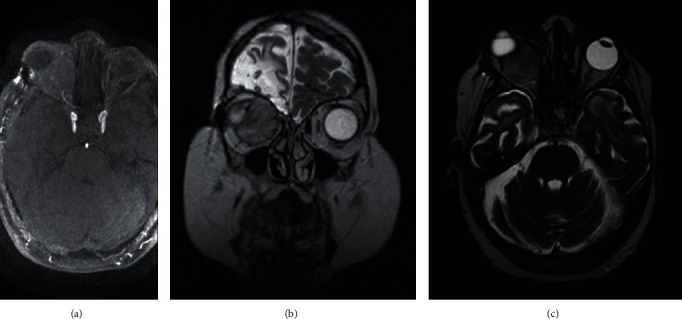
Case 4. (a) CT imaging with a great lesion, hyperdense morphology appears in the right intraconal region. The lesion imprints on the back of the globe, as well as the optic nerve and internal rectus muscle, with close contact with the external rectus muscle. (b, c) MR imaging, coronal view, presents lesion isodensity in T1- and T2-weighted sequences.
